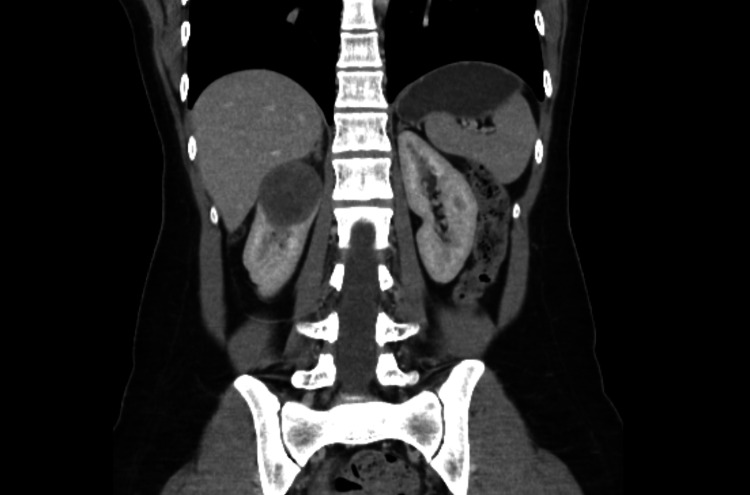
Coronal thoracoabdominopelvic CT showing a 45 x 40 mm complex cystic lesion consistent with a haemorrhagic cyst CT: computed tomography
